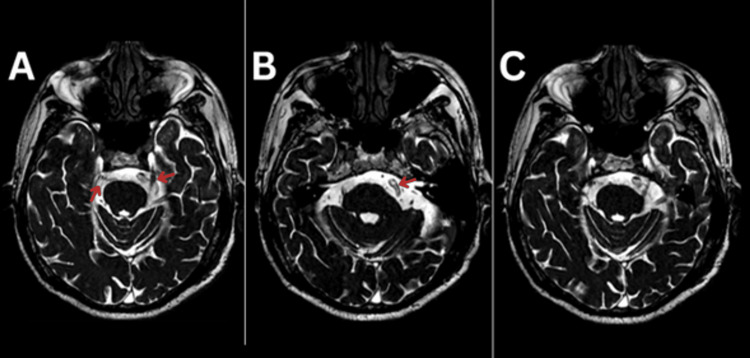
Preoperative MRI of contrast-enhanced T2-weighted fast imaging employing steady-state acquisition (FIESTA) sequence A) Bilateral neurovascular conflict; B) Visible dolichoectatic artery; C) Multiple neurovascular conflicts.
